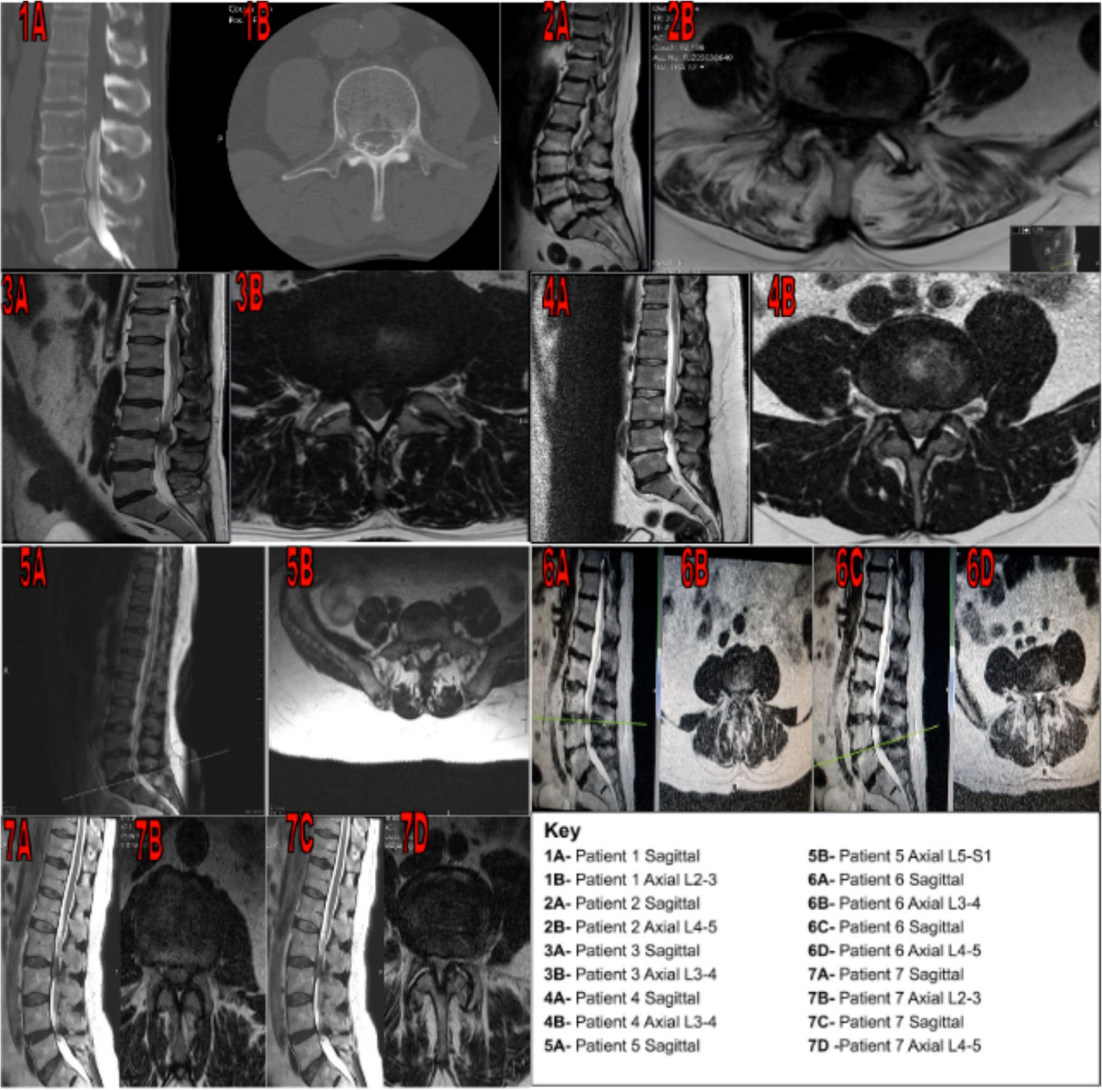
Sagittal and axial imaging views of the seven patients with acute bilateral foot drop. In patient 1, where an MRI was contra-indicated, a myelogram was performed. The levels affected ranged from L2/3 to L5/S1. The most commonly affected level was L3/4 in three out of the seven patients
